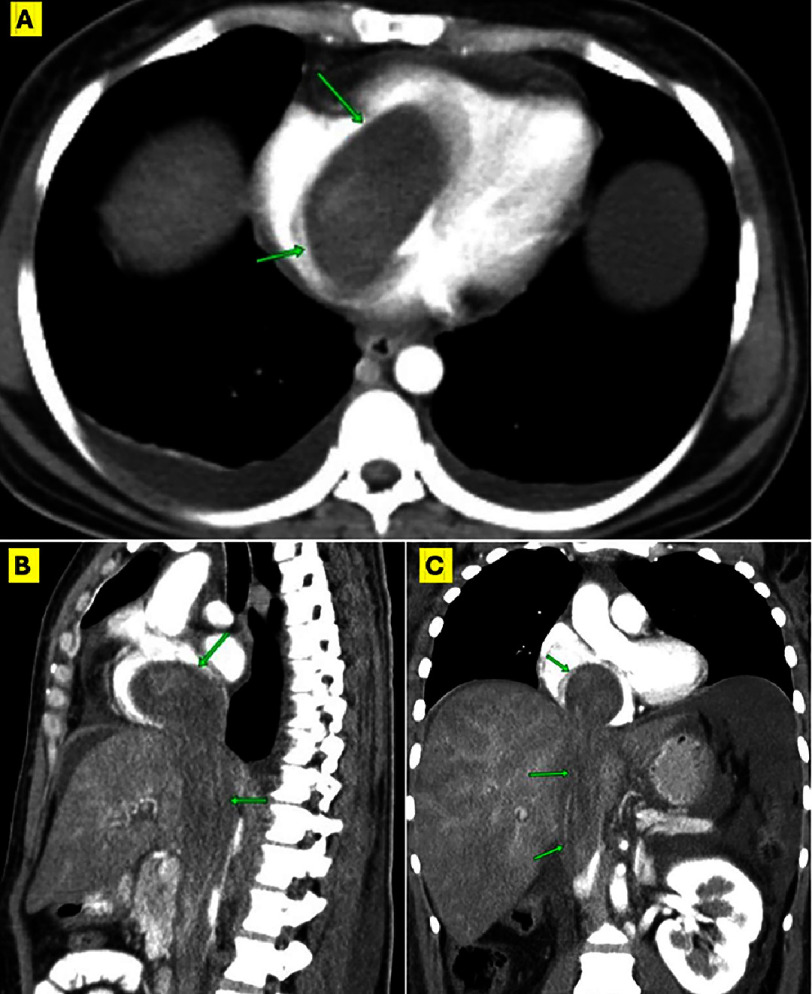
Pre-operative thoracoabdominal CT with contrast. (A) Contrast-enhanced CT showing a 4-chamber view and the large mass (green arrows) prolapsing from the RA to the RV. (B) Sagittal- and (C) coronal CT views showing the mass extending from the IVC to the RA. CT, Computed tomography; Other abbreviations as in Figure 1.
